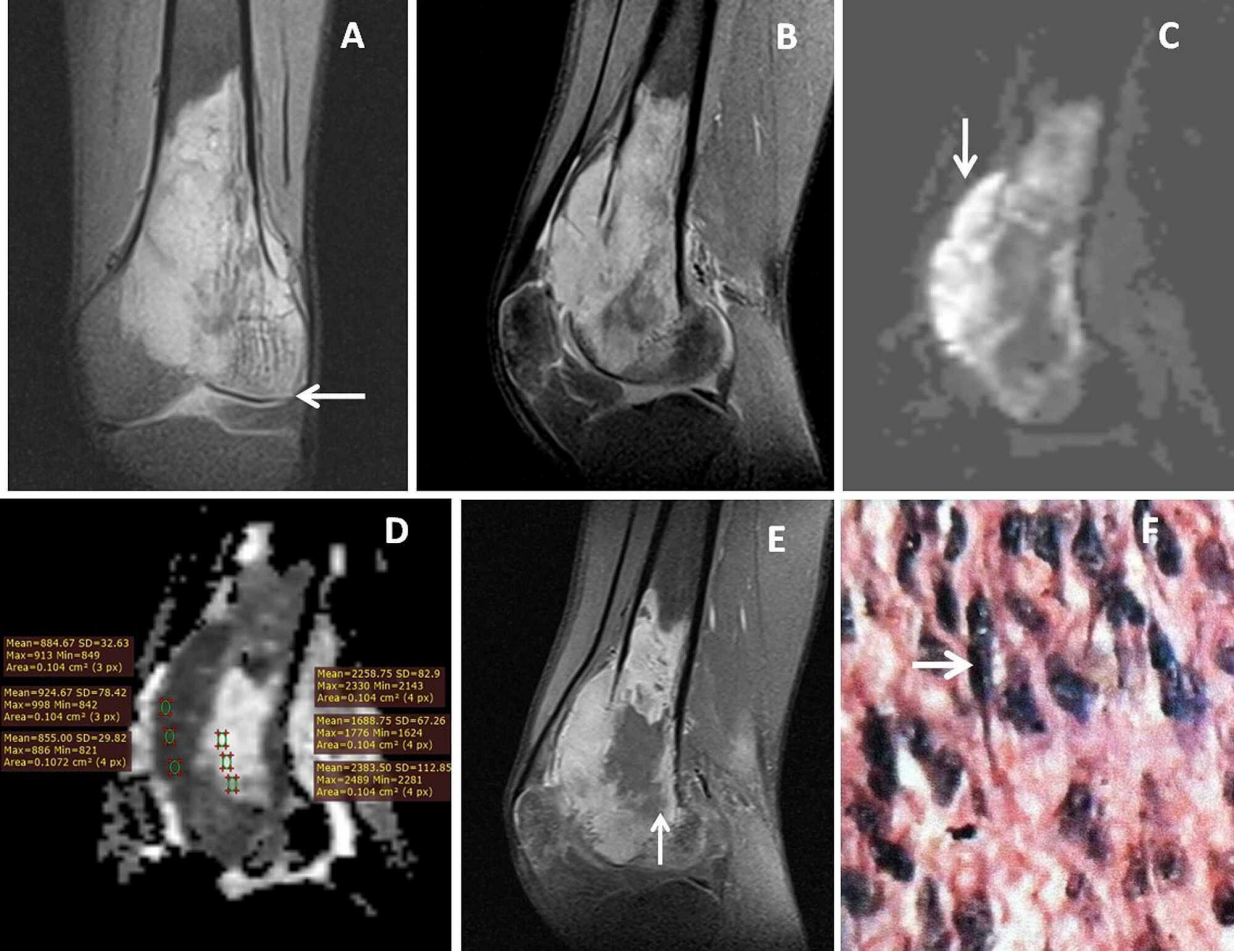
Distal femoral fibrosarcoma Twenty-year-old male had swelling around the left knee joint. Coronal and sagittal fat-suppressed proton density (PDFS) image (A & B) showed eccentric hyperintensities in distal meta-diaphyseal region of femur with epiphyseal extension into lateral femoral condyle ( ← arrow) with destruction of cortices. Sagittal diffusion-weighted imaging (DWI) image (C) with b value 1000s/mm2 showed irregular patchy peripheral diffusion restrictions (↓arrow) where apparent diffusion coefficient (ADC) image (D) showed low ADC value in peripheral and high in central portion of the tumor. Post-gadolinium image (E) showed heterogeneous enhancement of the mass with central necrosis (↑arrow). 40X HPE image (F) showed highly pleomorphic cells ( →arrow) with features of fibrosarcoma.
